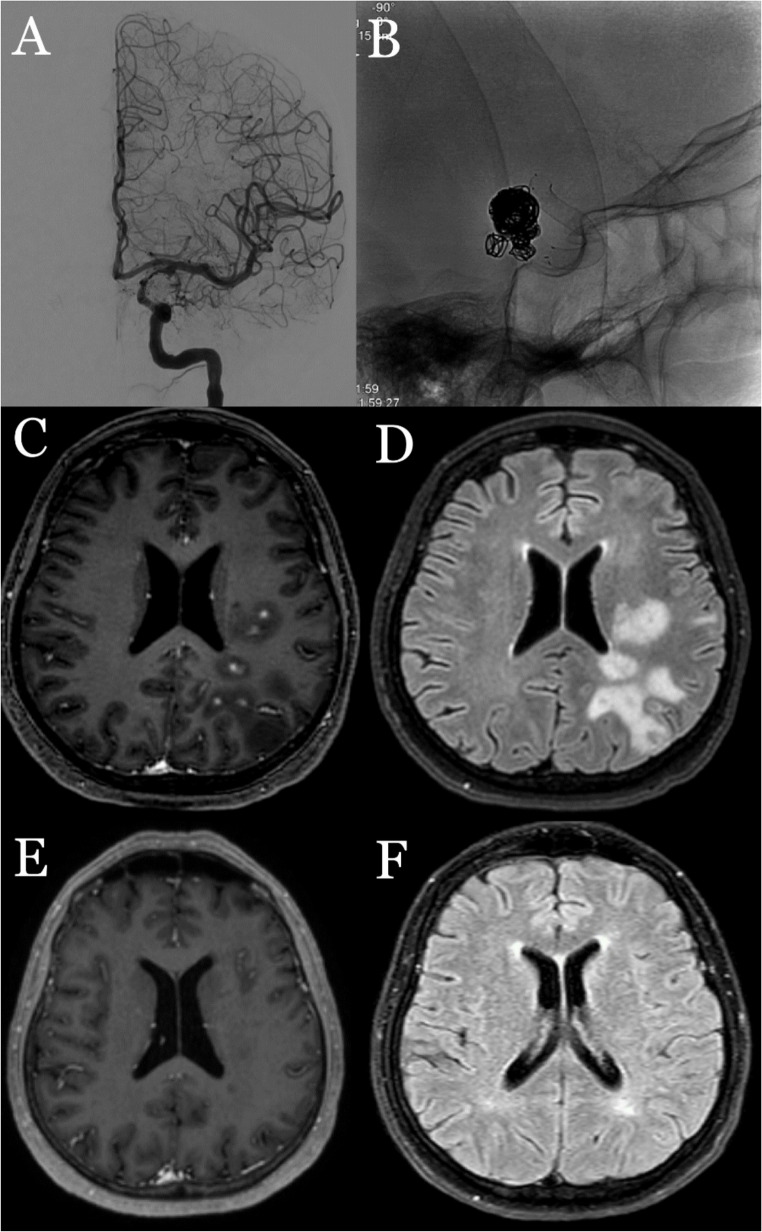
(A,B) Endovascular embolization with a flow-diverter stent (Derivo 4.5x20mm) and coil performed for two aneurysms in the left ICA. (C, D) MRI obtained 2.5 weeks post-procedure shows nodular lesions with contrast enhancement in the left hemisphere, surrounded by areas of vasogenic edema. (E, F) Follow-up MRI after treatment demonstrates regression of the NICE lesions and the surrounding vasogenic edema
